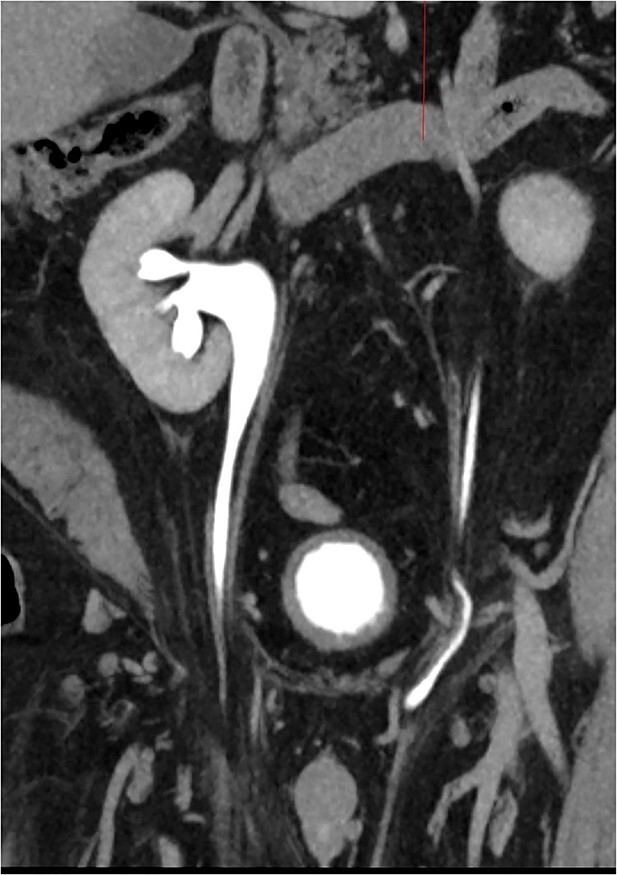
CT Urogram with bilateral ureteroinguinal scrotal hernia. Maximum intensity projection image has been reformatted to highlight both ureters crossing the inguinal canals.
